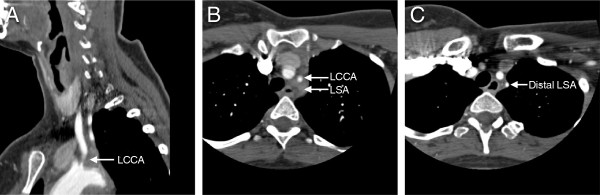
CTAs three months before CVA. Oblique lateral MPR view CT angiogram of the neck showing a thrombosed left common carotid artery (LCCA) with a high-grade stenosis at the origin of the common carotid, 1 cm in length (A). Transverse CTA just above the origin of the great vessels revealing complete occlusion of the left proximal subclavian artery (LSA) (B), with flow to the distal left subclavian artery provided by the left vertebral artery, indicative of subclavian steal (C).
